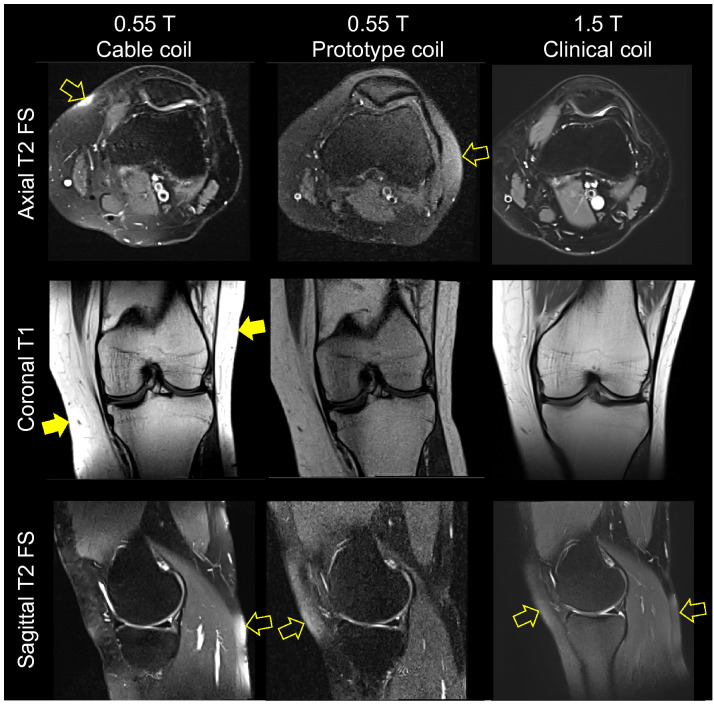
Turbo spin echo MR images from a 28-year-old woman acquired with the 0.55 T 6-channel cable coil (left column), 0.55 T prototype coil (middle column), and 1.5 T clinical coil (right column). The cable coil images have peripheral signal saturation (solid arrows). Images acquired with all coils had heterogeneous fat suppression (hollow arrows).
